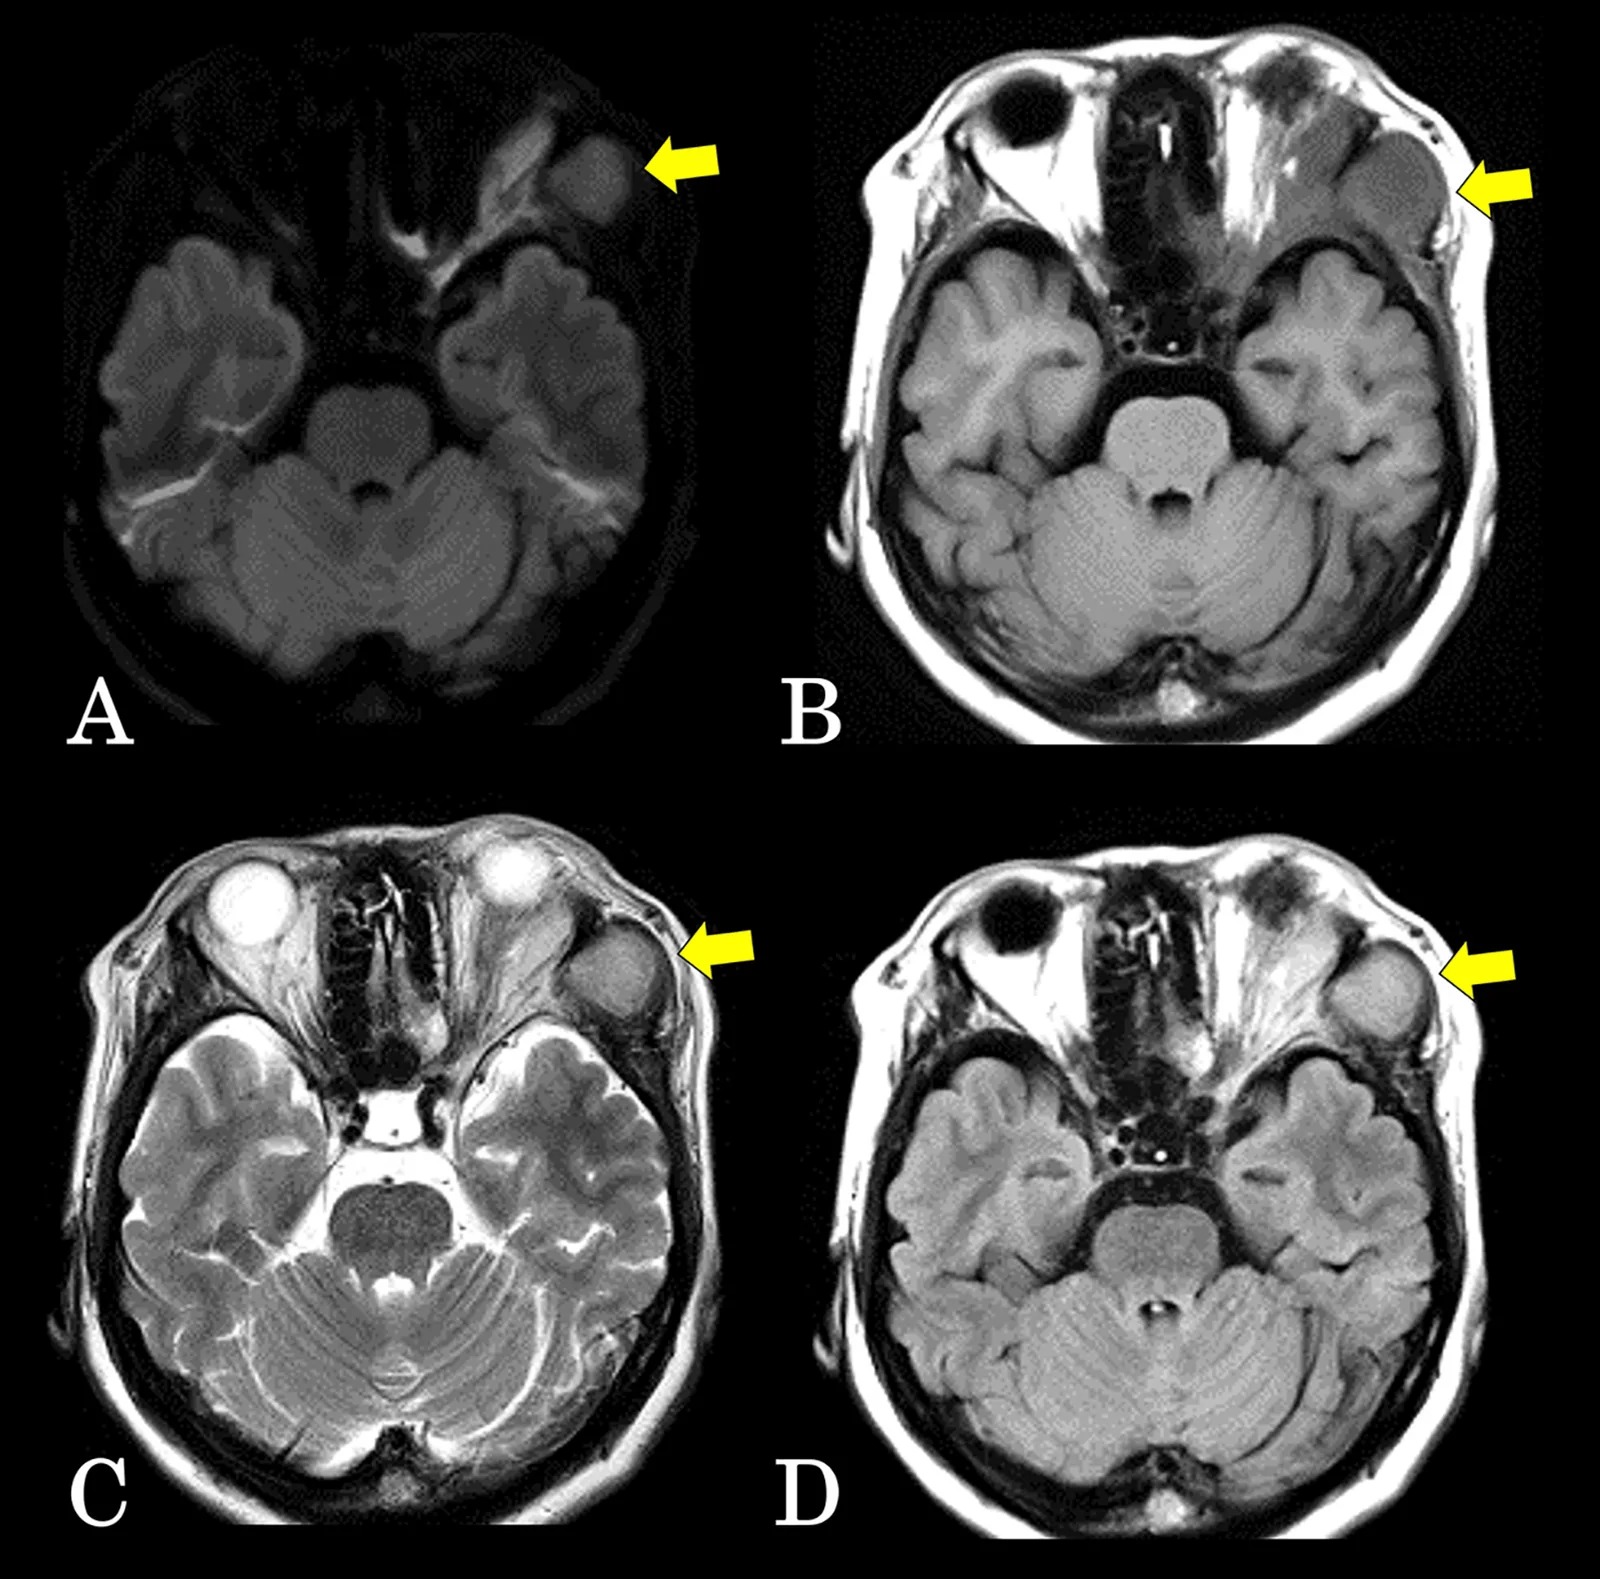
Magnetic resonance imaging findings (non-contrast). Arrows indicate the tumor. (A) Diffusion-weighted imaging showing no apparent diffusion restriction. (B) T1-weighted image demonstrating an iso- to slightly hypointense signal intensity. (C) T2-weighted image showing a slightly hyperintense signal. (D) Fluid-attenuated inversion recovery (FLAIR) image demonstrating a mildly increased signal intensity without surrounding edema.
